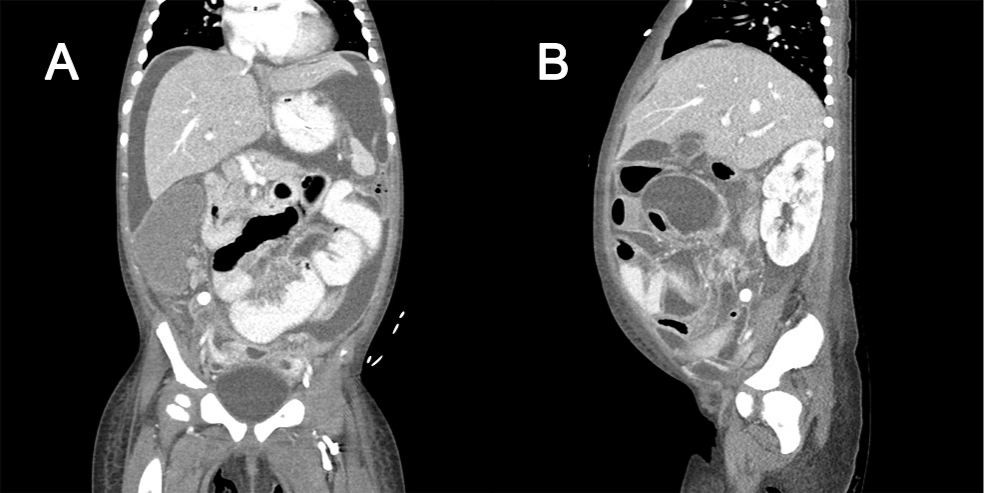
Computed tomography of the abdomen of Patient 3. Coronal plane image (A) demonstrating large amount of perihepatic intraperitoneal fluid and diffuse peritonitis. Sagittal plane image (B) demonstrating subdiaphragmatic fluid collections and appendicolith.
